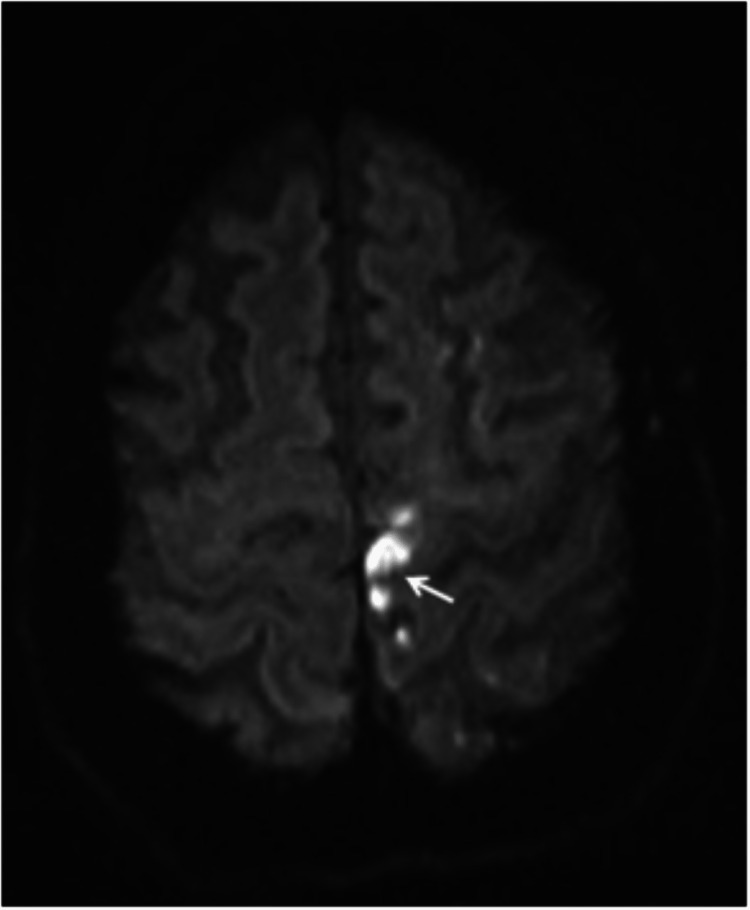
Diffusion-weighted MRI showing a left internal fronto-parietal cortical ischemic lesion (arrow) MRI, magnetic resonance imaging
